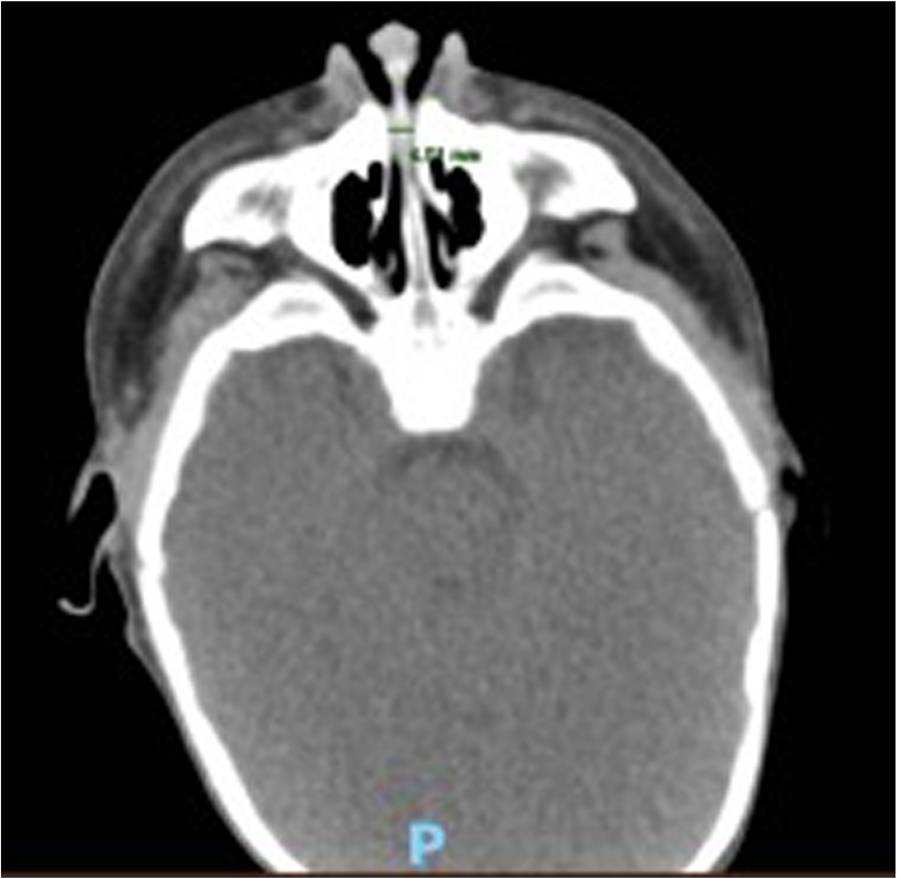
Preoperative head CT revealed pyriform aperture stenosis. The measurement was taken at the axial level of the inferior meatus, as recommended by radiological guidelines
